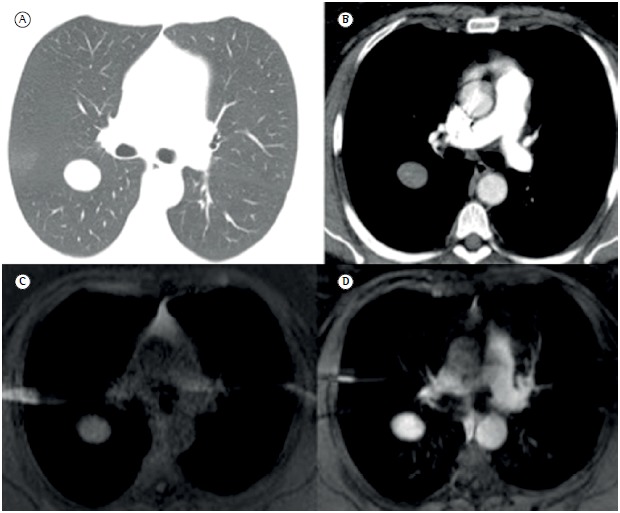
CT scans and magnetic resonance imaging of the chest. In A and B, chest CT
